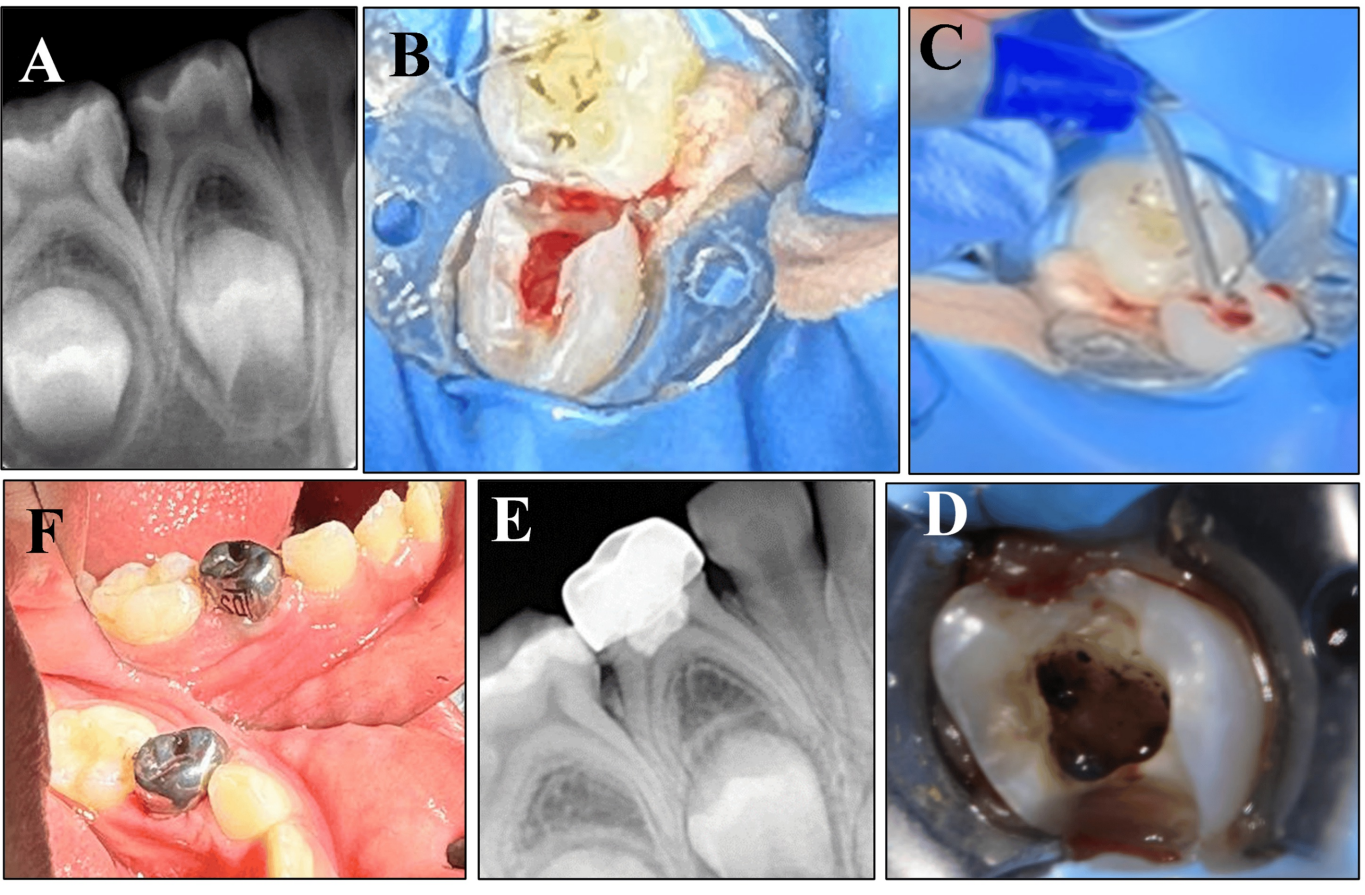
Sequence for the pulpotomy procedure using a diode laser. (A) Preoperative periapical radiograph of a first primary molar showing an extensive caries lesion on the distal side of the tooth. (B) Clinical removal of the pulp chamber roof and subsequent pulp exposure and pulp amputation. (C) and (D) Hemostasis of the pulpotomy sites using the diode laser. (E) Periapical radiograph showing the final restoration of the tooth. (F) Clinical photograph of the restored first primary molar.
